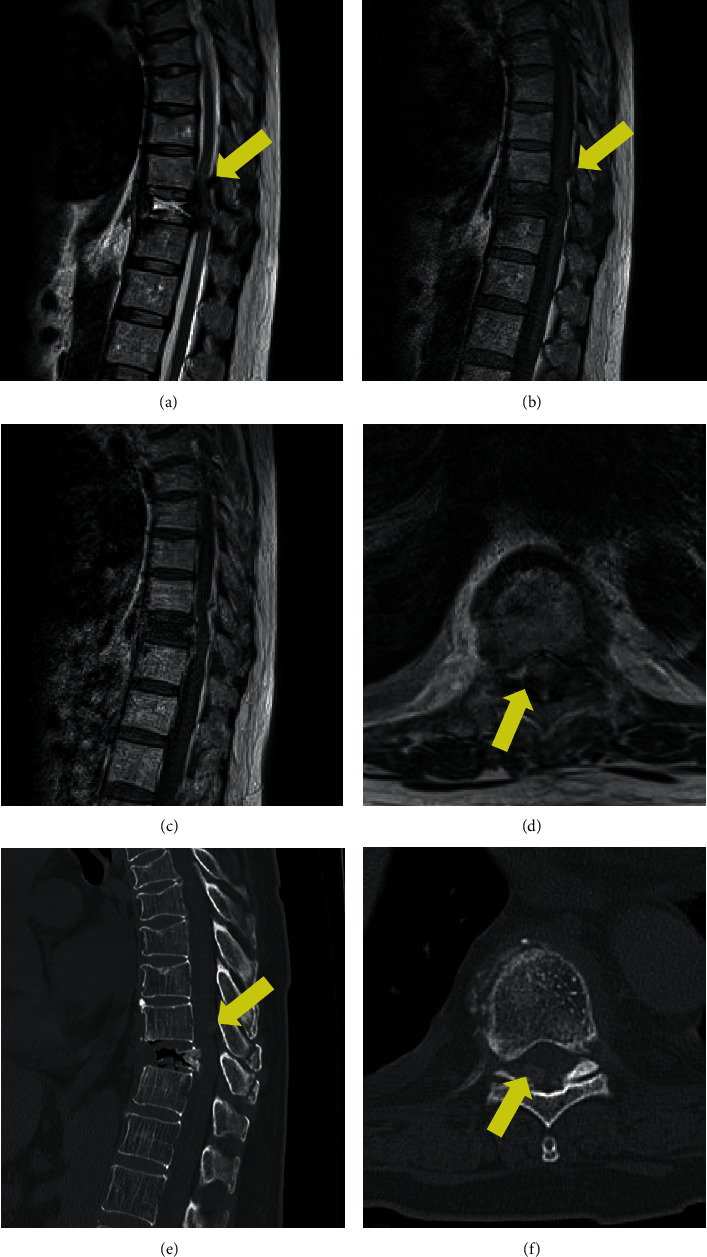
(a) T2 sagittal image showing a growing extradural mass (yellow arrow) at T9 level. (b) T1 sagittal image. (c) T1-enhanced sagittal image. (d) T2 axial image. (e) Computed tomography (CT) sagittal image demonstrating burst fracture of T10 vertebral body and slightly high-intensity mass at T9 level (yellow arrow). (f) CT axial image demonstrating mass effect at the right facet joint (yellow arrow).
